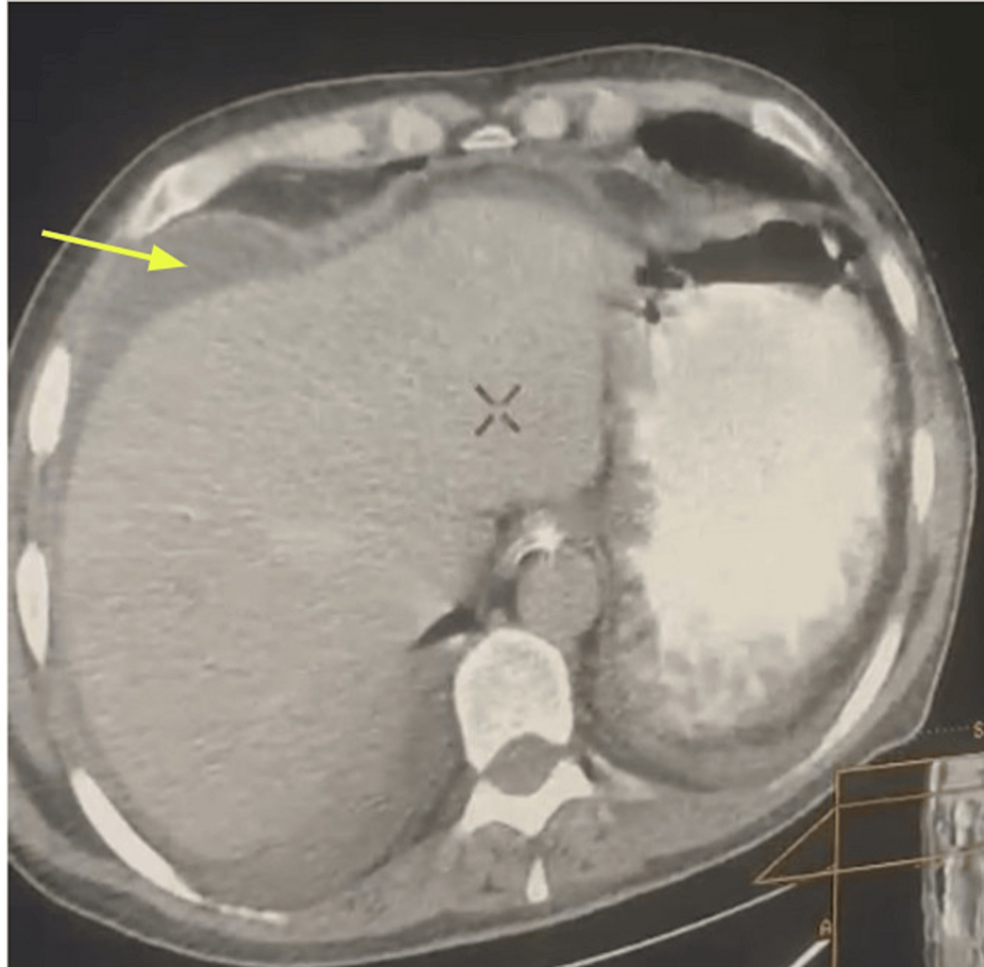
CT scan image revealed an intra-abdominal free fluid (yellow arrow).
